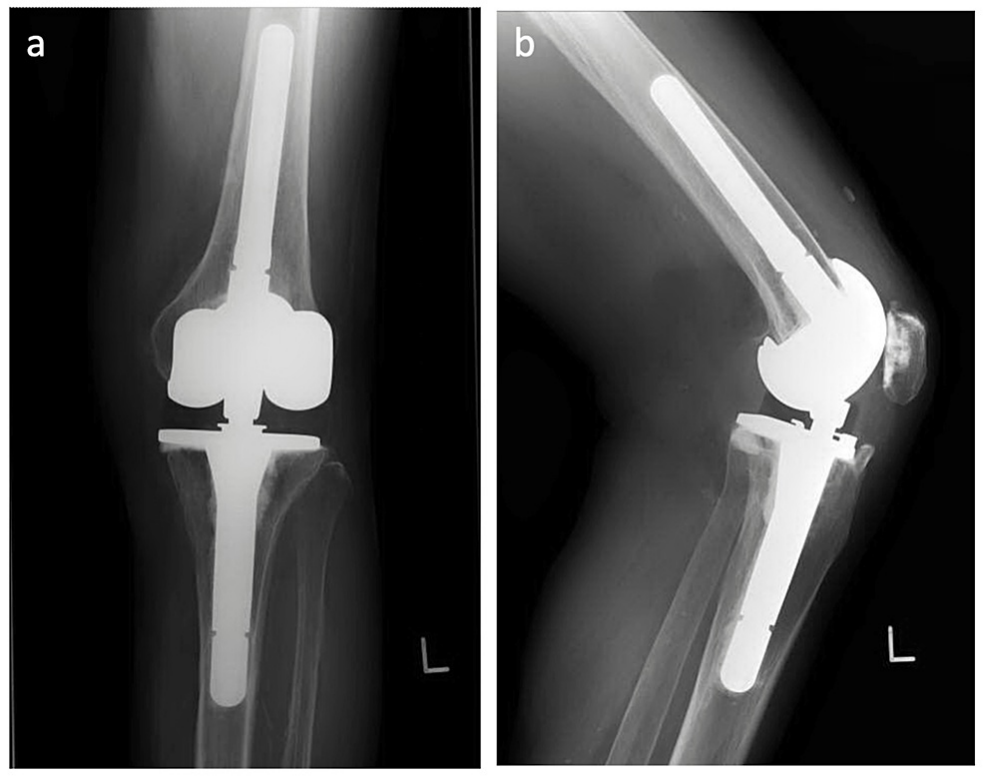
Postoperative radiographs. Anterior-posterior (a) and lateral radiographs (b) at 2 years after revision total knee arthroplasty.
